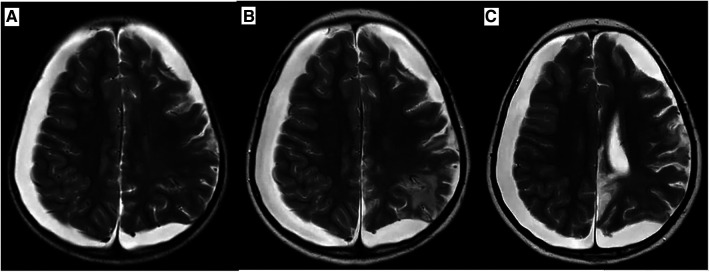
Axial T2 weighted Brain MR image during disease progression. (A) Brain MRI at first visit revealed atrophy of left hemisphere, subdural effusion, calcification foci, hemorrhagic region, ischemic region and vasculitis. (B) Brain MRI after one month of treatment which revealed the size of ischemic region increased. (C) Brain MRI after one and a half months of treatment which revealed medium amount of subdural effusion and size of ischemic region increased.
